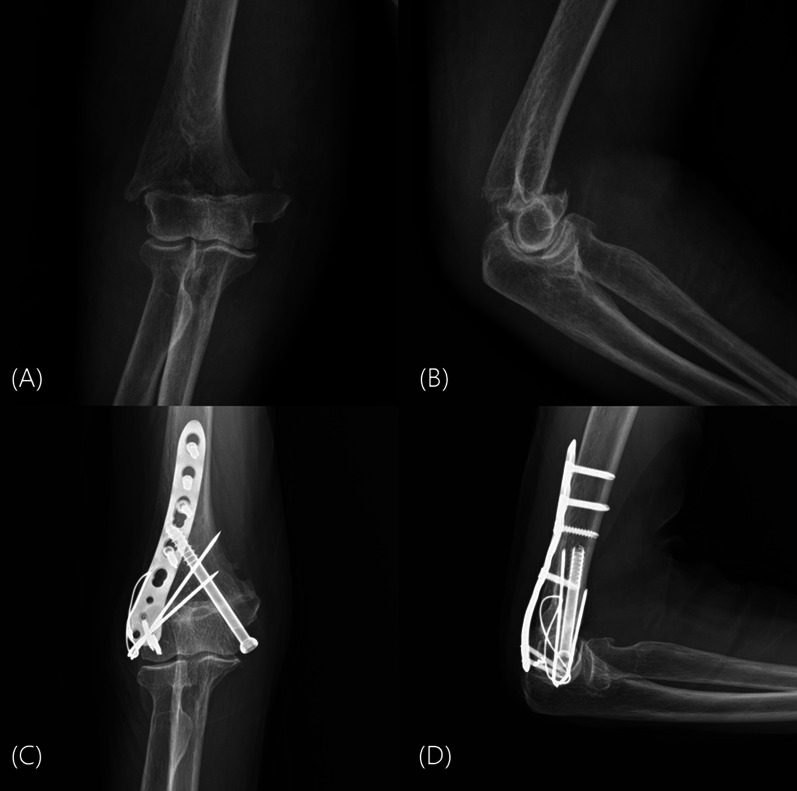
An 85-year-old female patient treated with single-plate fixation with a medial screw. A, B Preoperative radiographs showed an extra-articular distal humerus fracture with subsequent medial translation of the distal fragment. C, D Additional tension-band wiring was performed for lateral column stabilization. Postoperative radiographs obtained at final follow-up demonstrated bony union without any signs of displacement
